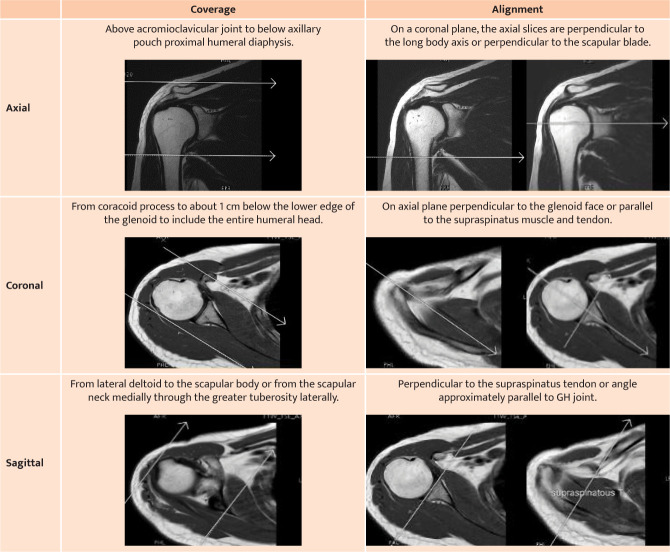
The level 2 category focuses on the sequences protocol that controls the signal intensity and image contrast of the tissues. As clinical requirements continue to evolve, imaging sequences are constantly being modified to address more specific clinical needs [11]. On average, MRI shoulder protocols consist of 4-5 sequences [12]. The ACR requires at least four mandatory sequences. These include two coronal oblique planes, one fluid-sensitive sequence with a suppressed fat signal (T2W FS), and another dark fluid sequence (T1W). The other two sequences are a proton density for axial (Long TR, Short TE PD) and a bright fluid for sagittal (T2W) [13].
